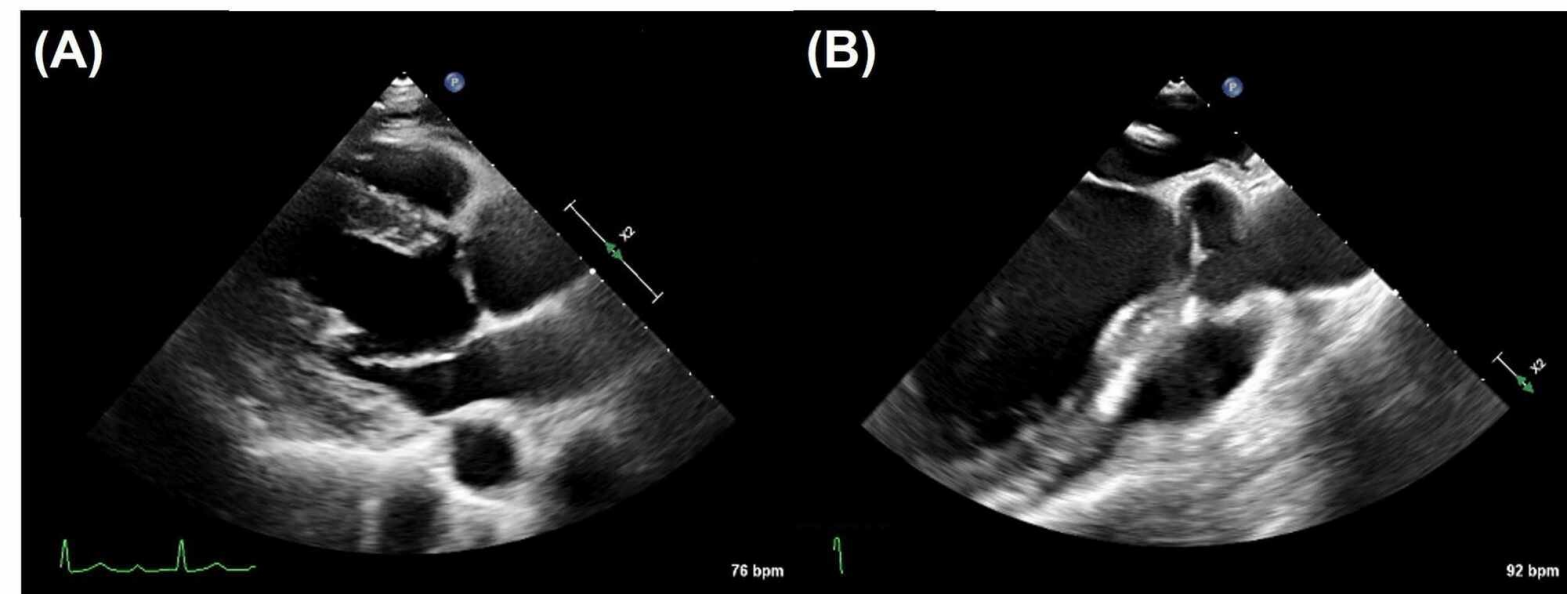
Transthoracic and transesophageal echocardiography Transthoracic (A) and transesophageal echocardiography (B) revealed no remarkable abnormalities suggesting infective endocarditis.
